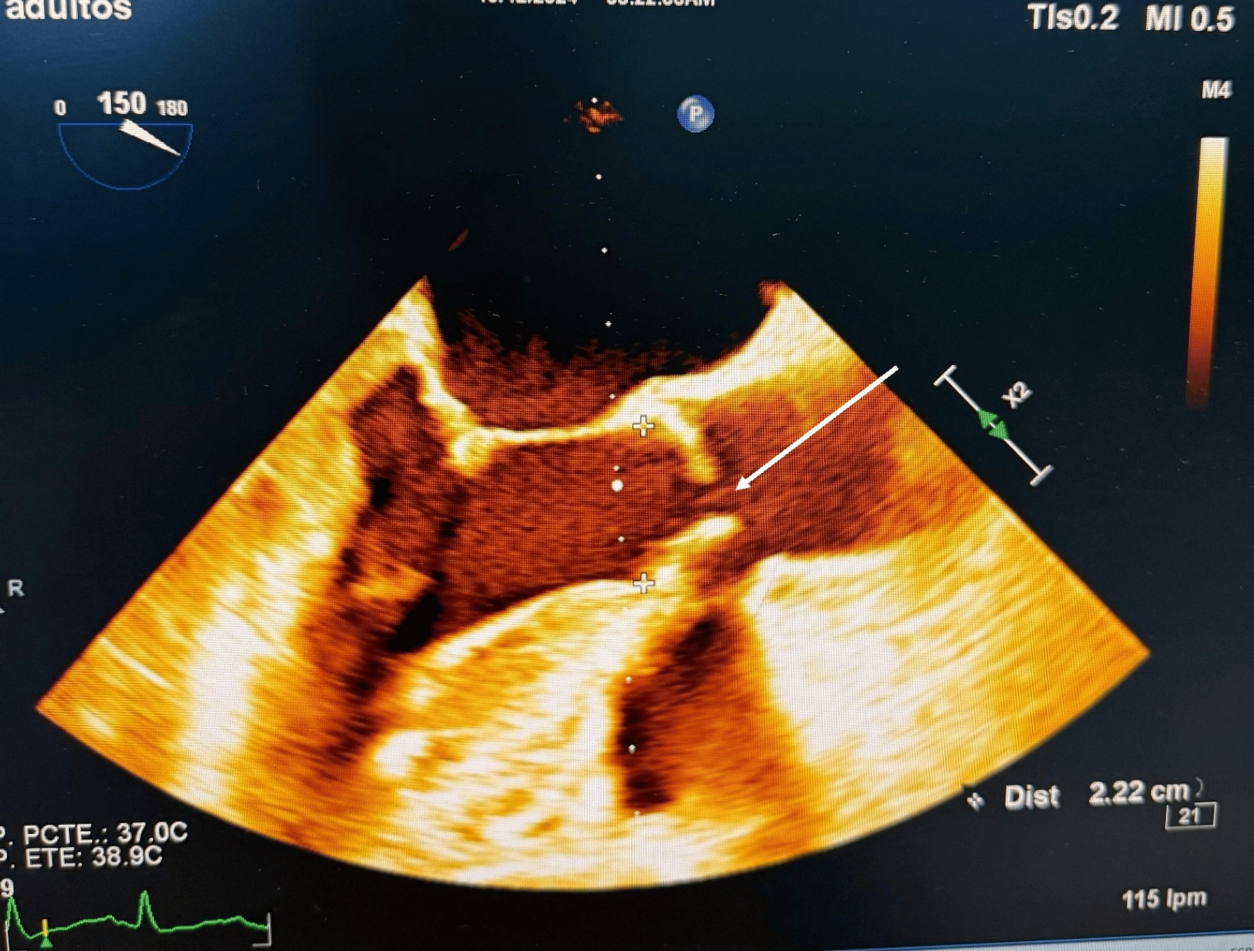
TEE mid-esophageal aortic valve long-axis view revealing severe aortic stenosis TEE: Transesophageal echocardiography
